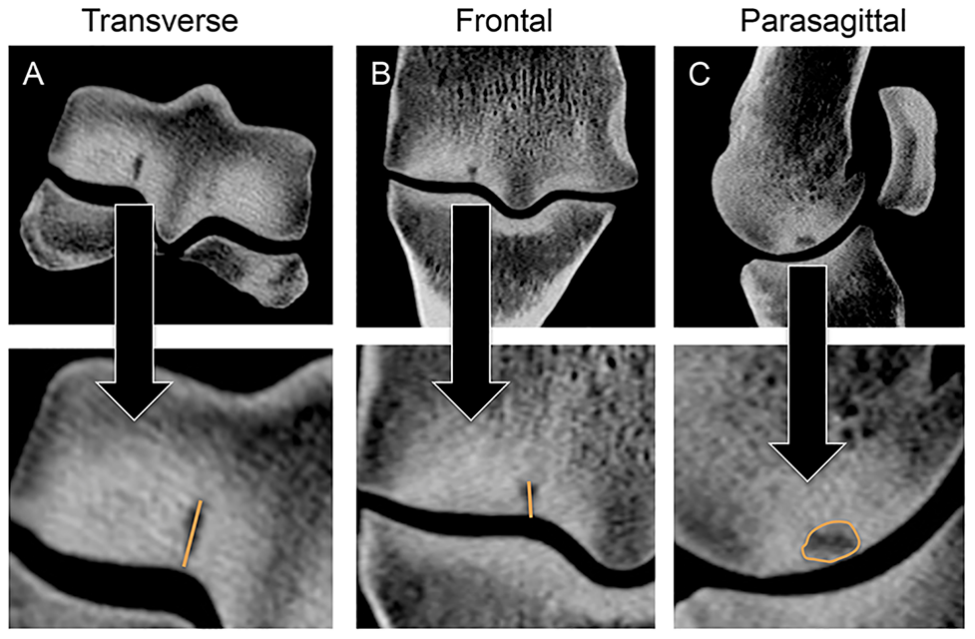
Subchondral fatigue crack dimensions were measured in transverse (A) and reconstructed frontal plane (B) computed tomography (CT) images. In addition, the area of the crack array was measured in the parasagittal plane (C).
